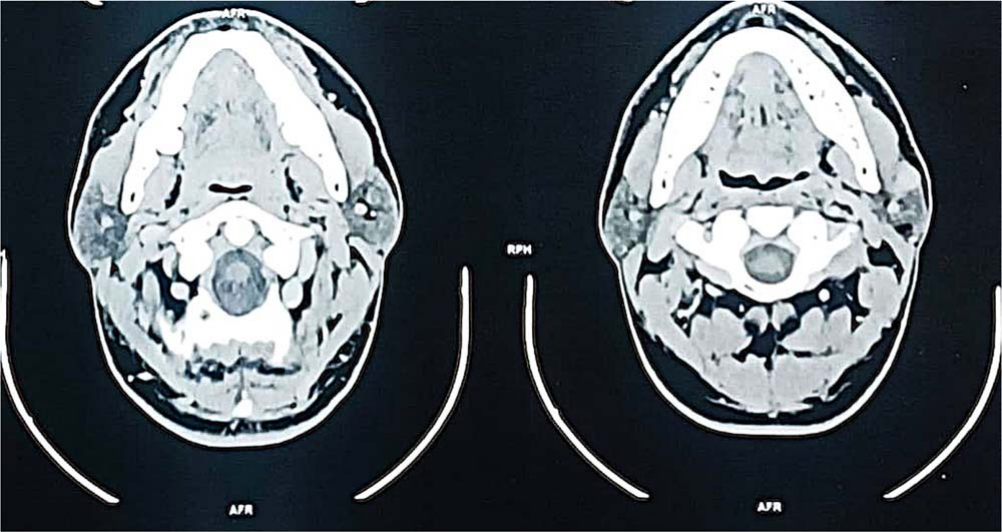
Axial contrast-enhanced CT scan demonstrating a well-defined, enhancing mass in the superficial lobe of the left parotid gland.
